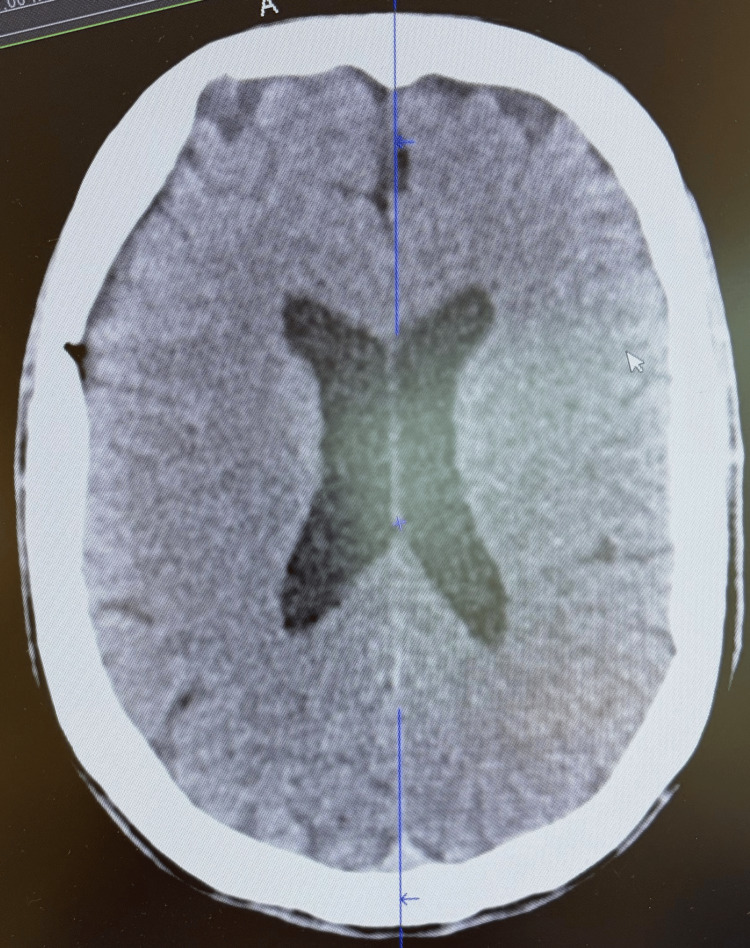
Non-contrast computed tomography (CT) of the head. Axial slice demonstrating no acute intracranial pathology, hemorrhage, or midline shift. The imaging was interpreted as normal, redirecting the diagnostic focus toward metabolic etiologies.
